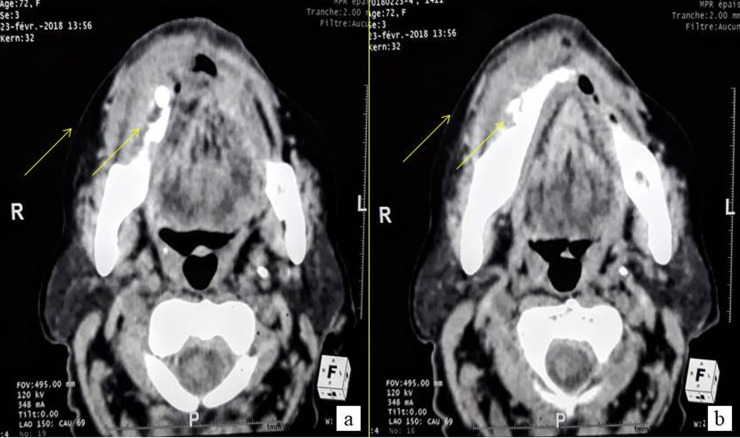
craniofacial CT-scan: axial sections, narrow window
